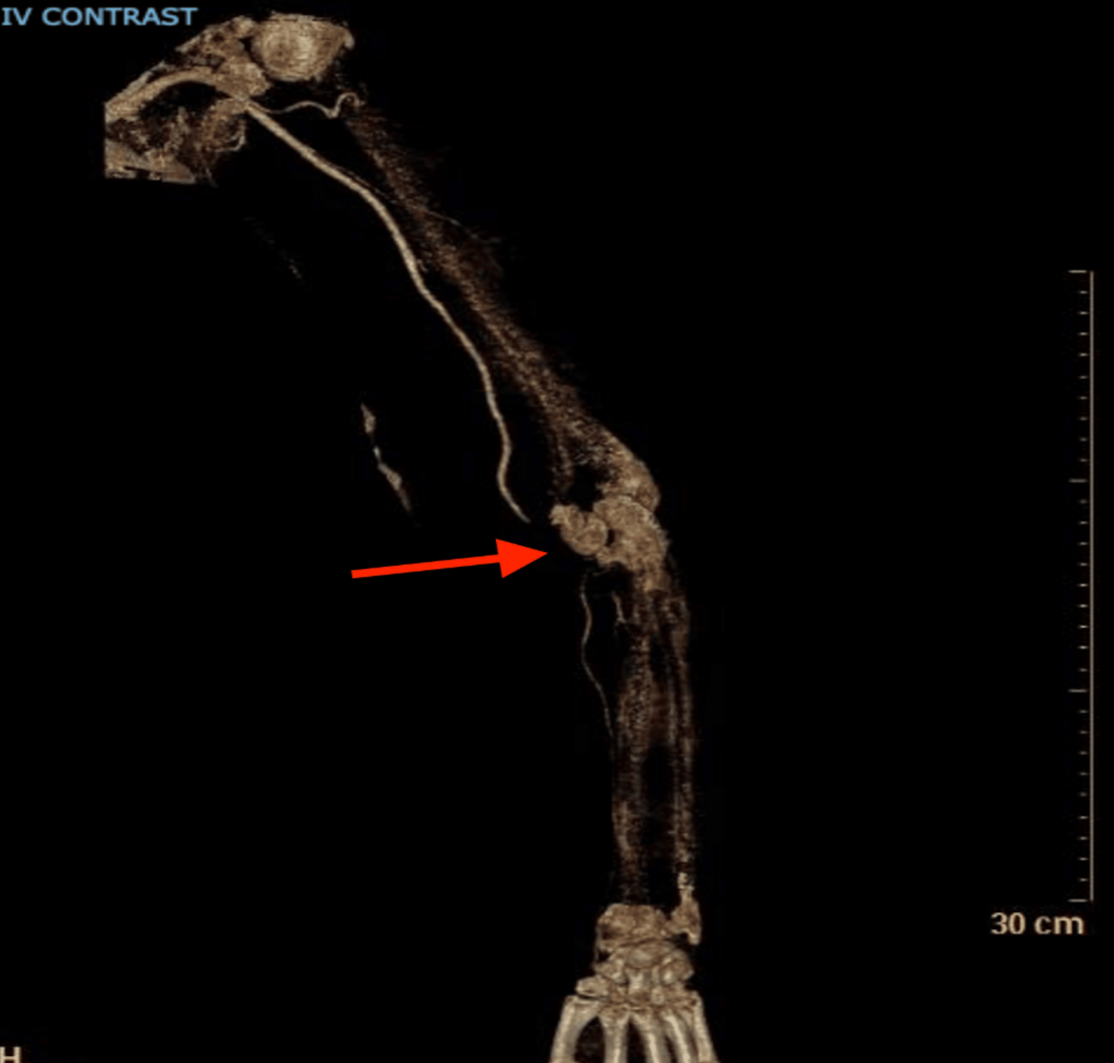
3D reconstruction from a contrast-enhanced CT angiogram The 3D reconstruction of the upper extremity from a contrast-enhanced CT angiogram reveals acute thrombosis of the brachial artery. There is an abrupt cutoff of contrast flow in the mid-to-distal brachial artery (red arrow), with absent distal opacification, consistent with complete occlusion. The affected vessel appears irregular, and there is no evidence of contrast extravasation. These findings are indicative of acute limb ischemia due to arterial thrombotic occlusion.
